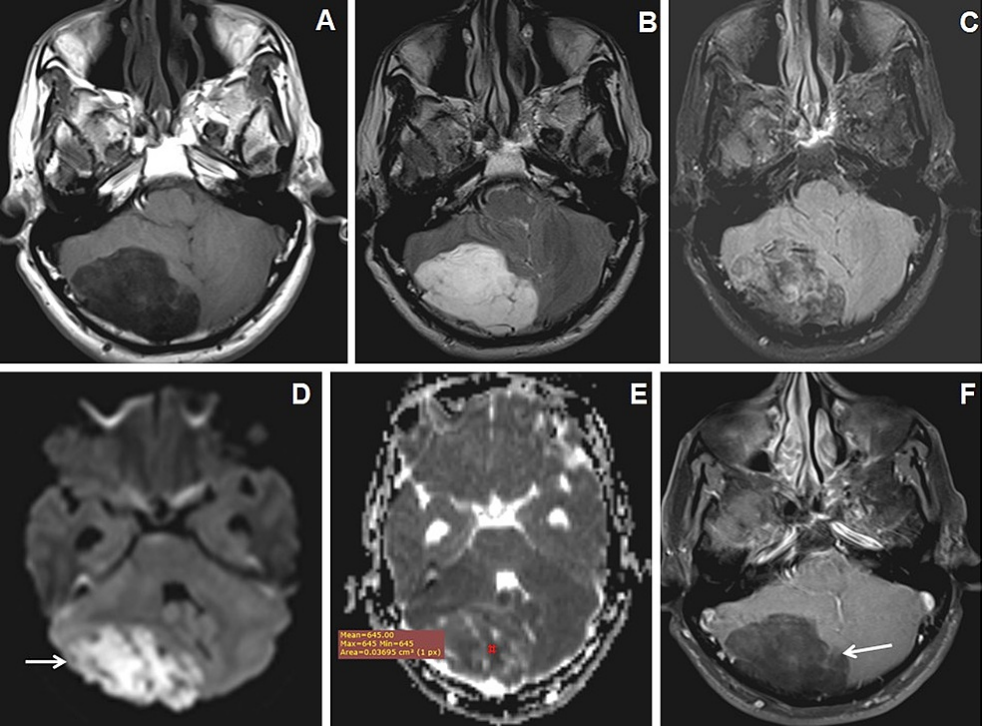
Cranial MRI of a 41-year-old male patient with headache due to epidermoid cyst. (A and B) Axial T1WI and T2WI images show an extra-axial heterogeneous appearing T1WI hypointense and T2WI hyperintense lesion over the right cerebellar convexity with intervening T2W hypointense septae. (C) FLAIR image shows incomplete suppression of the cyst. (D and E) Axial DWI and ADC map images show restricted diffusion (→arrow) with variable ADC values. (F) Axial T1W post-contrast image shows no enhancement (←arrow). FLAIR: fluid-attenuated inversion recovery; DWI: diffusion-weighted imaging; ADC: apparent diffusion coefficient
